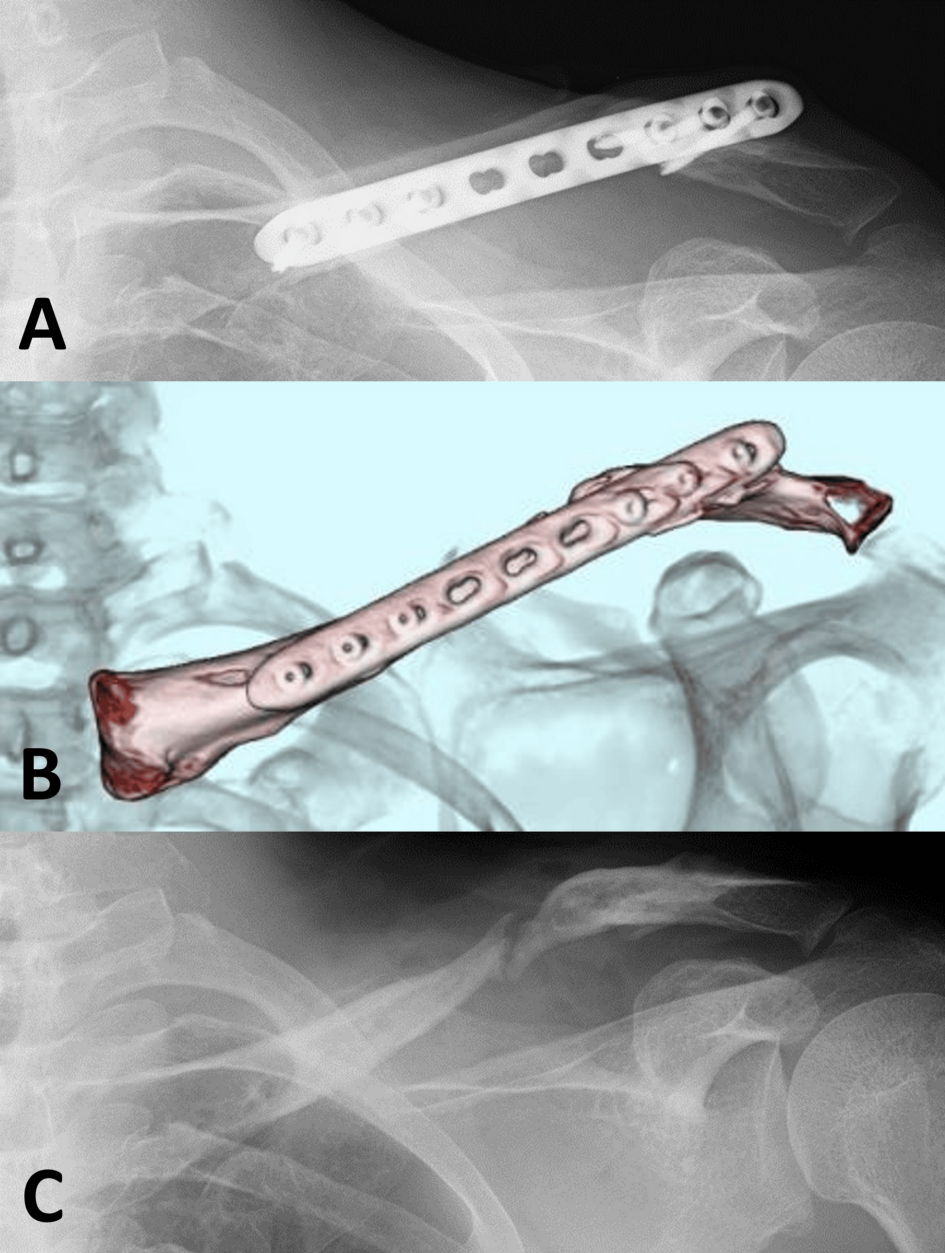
Nonunion after initial surgery X-ray imaging revealed protrusion of the distal end of the plate toward the skin (A), and three-dimensional computed tomography suggested a possible loosening of the distal screws (B) at one and a half years post-injury. An X-ray taken after plate removal revealed atrophic nonunion (C).
